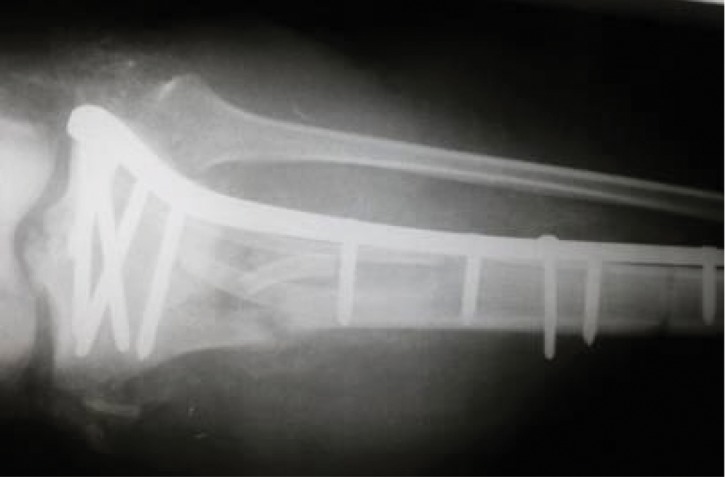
X-ray of the tibia with the rib trisected and used to fill the bone defect.
